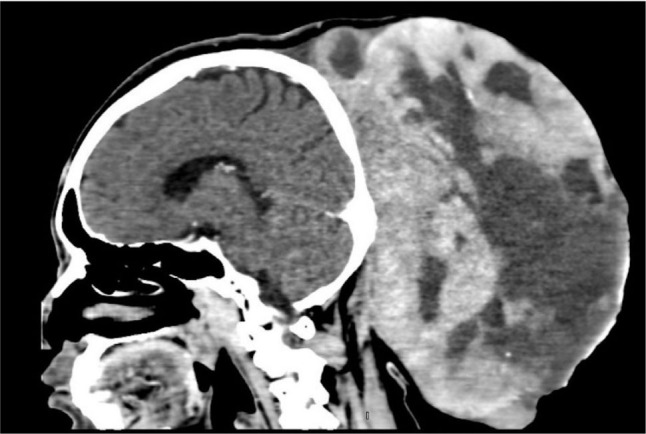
CT scan of brain with contrast (sagittal view).
